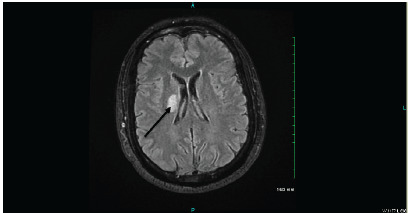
Axial T2-weighted magnetic resonance imaging (MRI) image showing hyperintensity (arrow) in the right caudate nucleus, consistent with an acute ischemic stroke.
